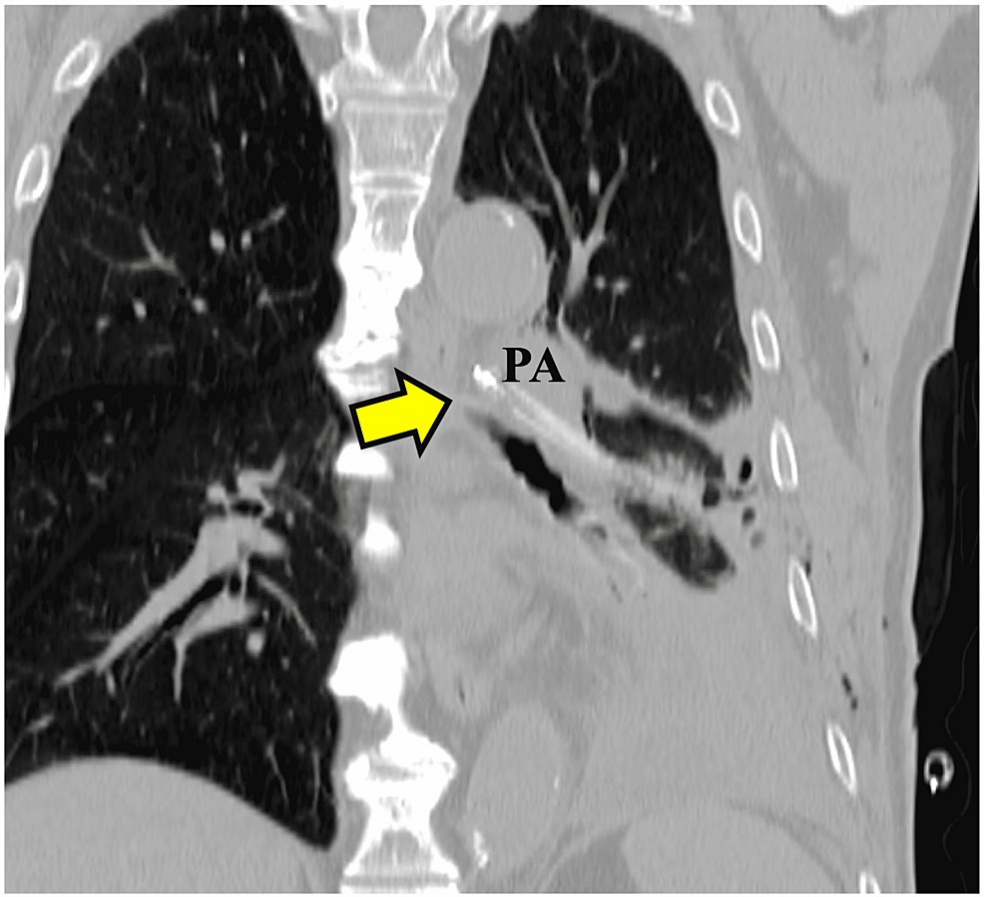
Preoperative coronal computed tomography The chest tube has migrated approximately 10 cm into the left lower lobe (arrow). No massive intrapulmonary hemorrhage, pneumothorax, or pneumomediastinum is observed. PA: pulmonary artery
